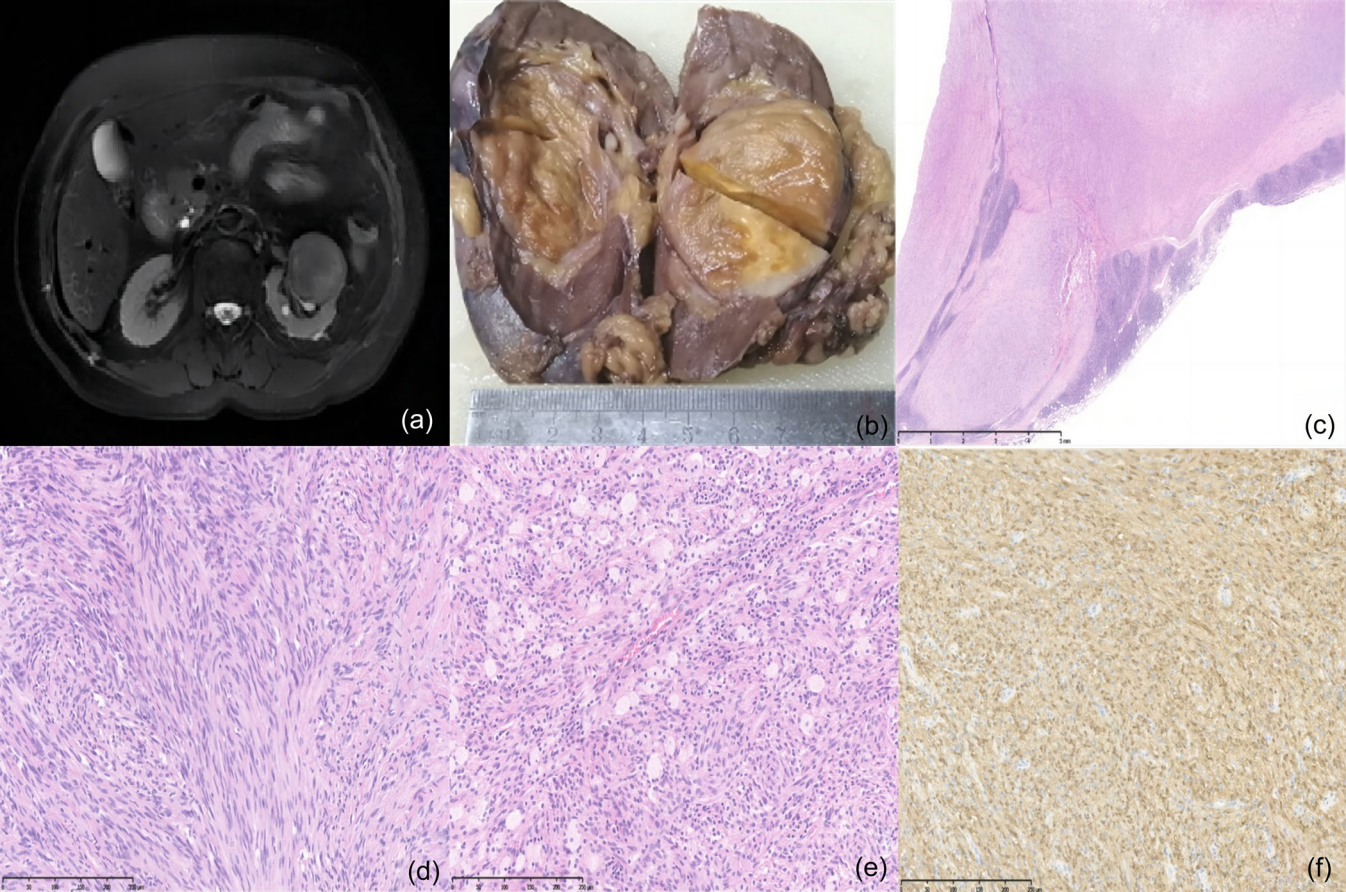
(a) A computed tomography image showing a solid nodule in the left kidney. (b) Grossly, the sectioned tumor appears yellow to brown with a clear margin. (c) The tumor appears multinodular and has a fibrous capsule with a lymphatic sheath outside the capsule. (d) The spindle tumor cells are arranged in a strip structure. (e) Foam cells and lymphocytes are found in the thick stromal. (f) The tumor cells exhibit immunopositivity for S100.
